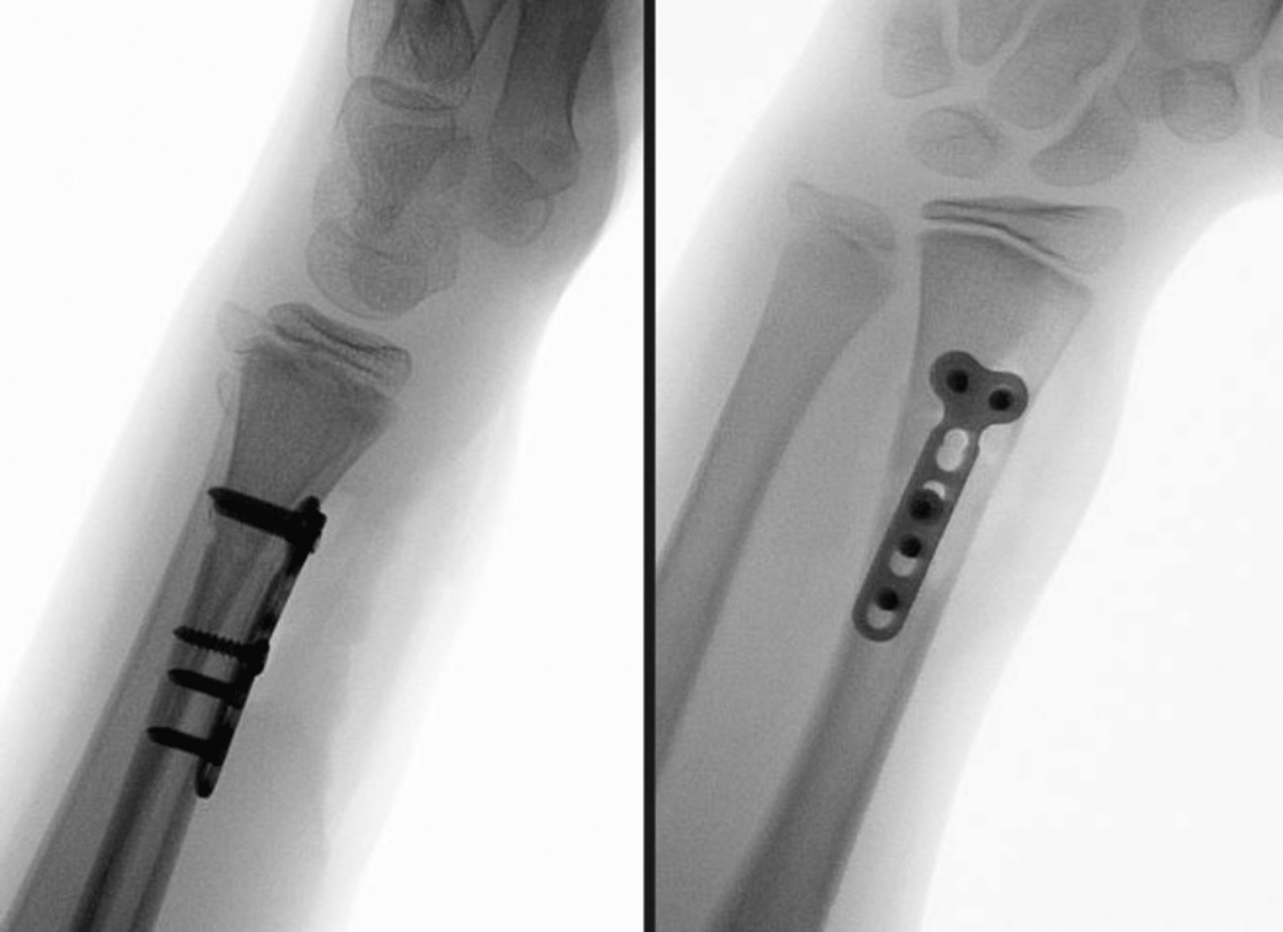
Intraoperative fluoroscopy X-rays of the right forearm (lateral and anteroposterior views) with volar locking plate
